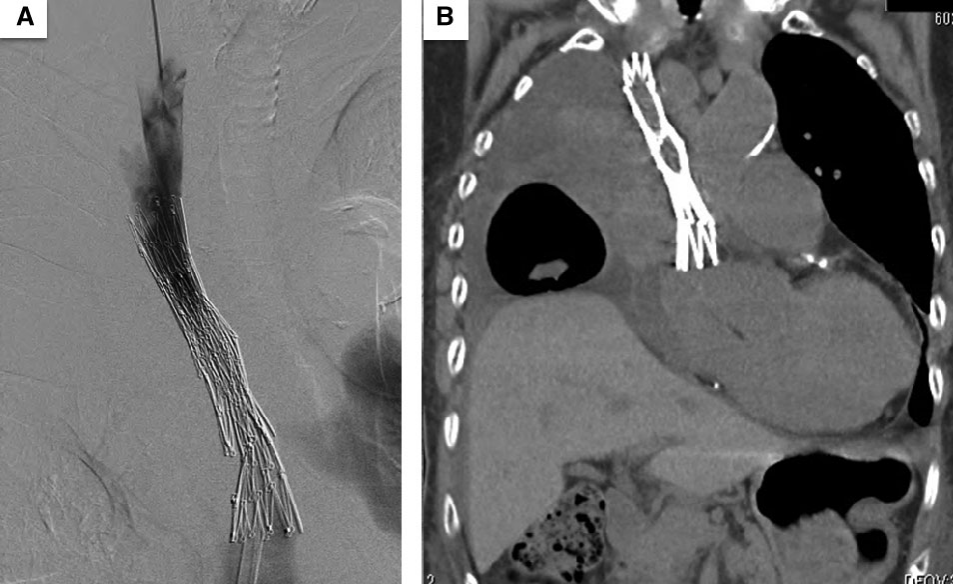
An angiograph (A) and computed tomography (CT) scan (B) of the post‐stenting superior vena cava (SVC). (A) An additional SVC stent was placed and post‐stenting venogram showed flow improvement in the SVC. (B) The distal end of the additional stent was located just proximal to the right atrium.
